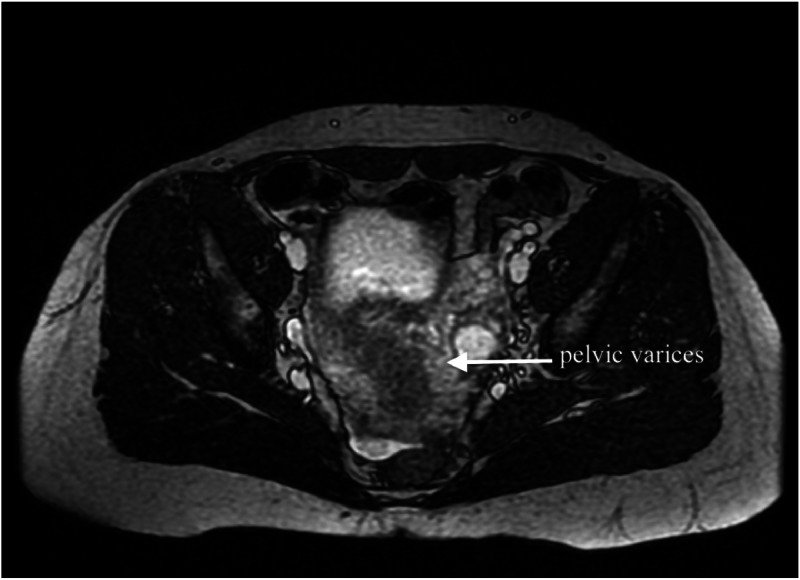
Axial T2-weighted MRI showing dilated parauterine veins (arrow) consistent with pelvic varicosities. These venous dilatations are characteristic findings of pelvic venous insufficiency in patients with chronic pelvic pain.
